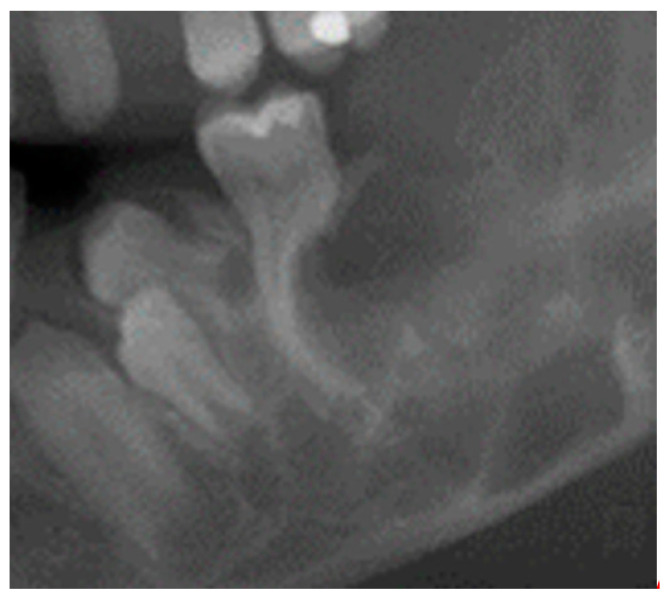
An X-ray showing root resorption in a cherubism patient after contact with osteoprotegerin (OPG).
